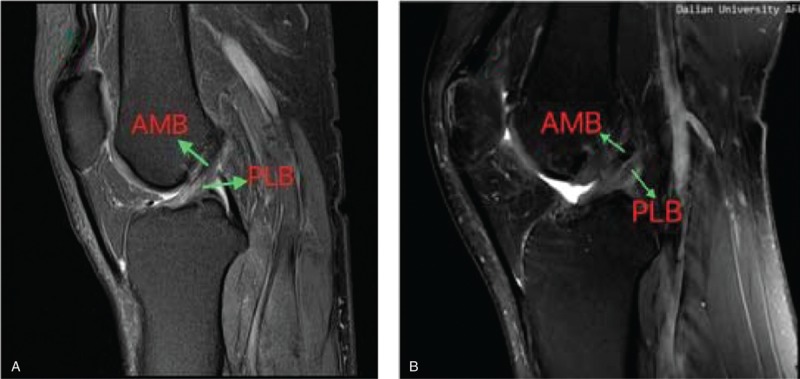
a: on oblique sagittal MRI, the 2 bundles were seen clearly, and near parallel with the knee extended (Healthy people);b: Under oblique sagittal MRI, the 2 bundles were seen clearly, and near parallel with the knee extended (postoperation). MRI = magnetic resonance imaging.
